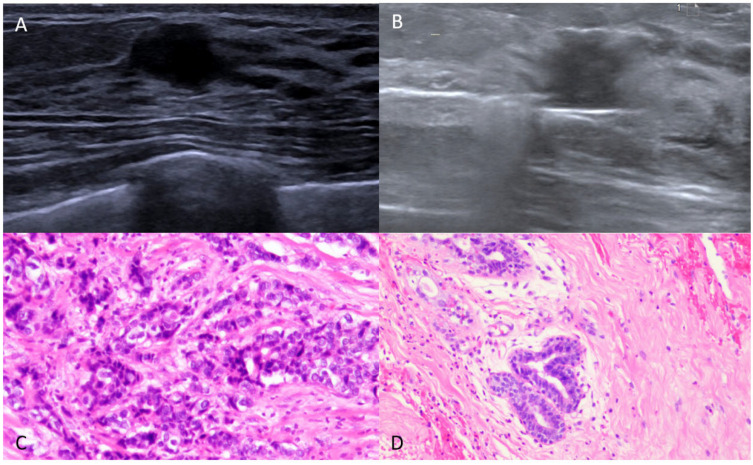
A 34-year-old lady with a hypo-echoic lesion in the left breast. (A) The lesion is irregular, with duct change. It was categorized as BI-RADS 4A. (B) Ultrasound-guided vacuum-assisted excision was performed. (C) Pathology showed invasive ductal carcinoma (grade III). (D) Post-surgery showed intermediate–high-grade ductal carcinoma in situ.
